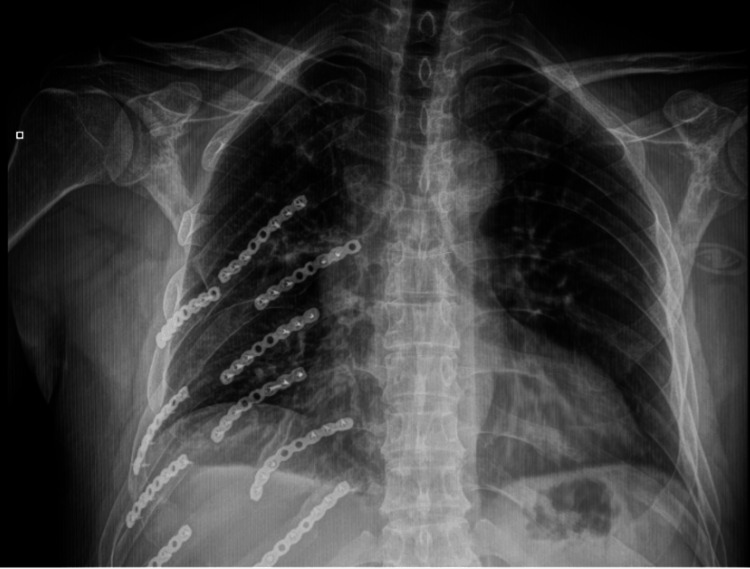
Follow-up posteroanterior chest radiograph taken two months after surgery. Posteroanterior chest X-ray showing improved bilateral lung aeration. Persistent perihilar infiltrates and basal atelectasis in the right lung are noted. Osteosynthesis material in the right rib cage is in the proper position.
